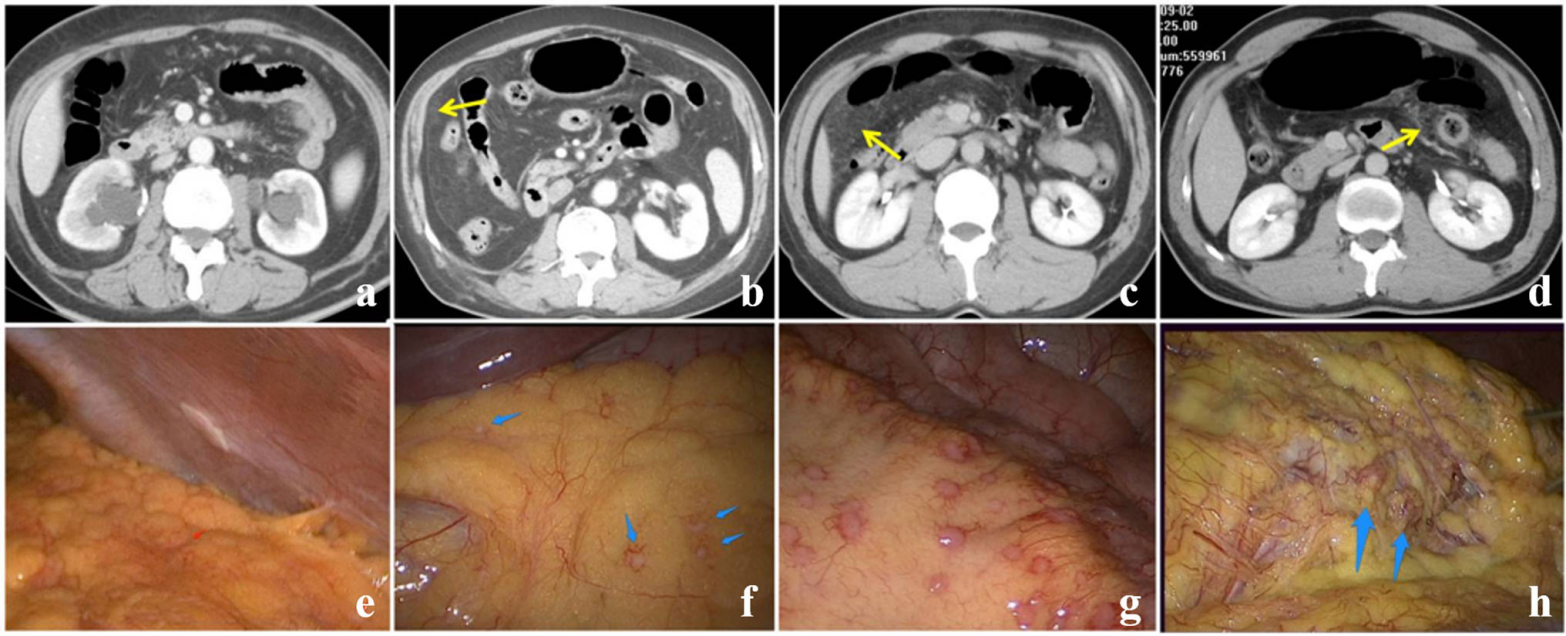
Occult peritoneal metastasis (OPM) score of free peritoneum. a OPM grade 0 (no abnormal signs). b OPM grade 1 (slightly increased fat density appearing as S-GGO). c OPM grade 2 (increased fat density with patchy S-GGO). d OPM grade 3 (obviously increased fat density with multiple strands and blurred small nodules). e–h Intraoperative screen shots of staging laparoscopy corresponding to the aforementioned computed tomography (CT) findings. The degree of metastasis is displayed from light to severe. S-GGO, smudge-like ground-glass opacity
